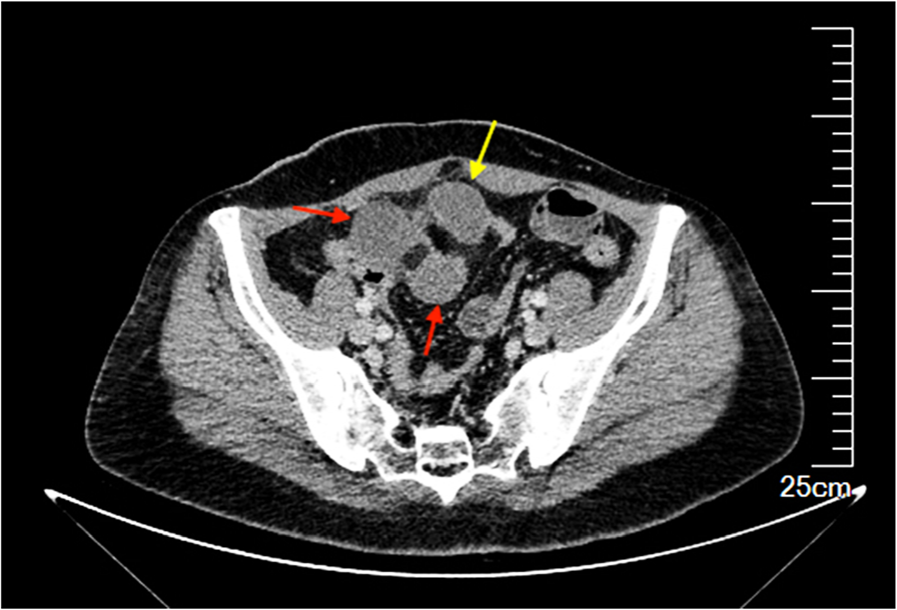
Computed tomography showing the thickened, dilated small bowel loop (red arrow) with surrounding stranding formed by Meckel diverticulum (yellow arrow)
